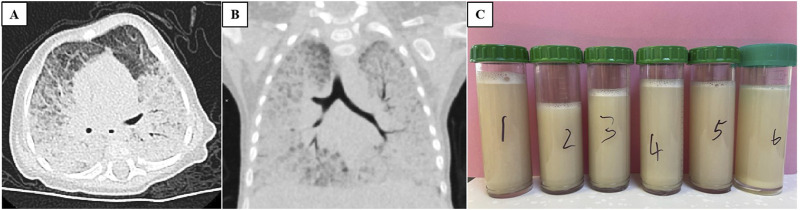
Chest CT scan ((A) transverse view and (B) coronal view) both showing widespread ground-glass opacities in both lungs, along with thickened interlobular septa. (C) Bronchoalveolar lavage fluid appeared milky white.
